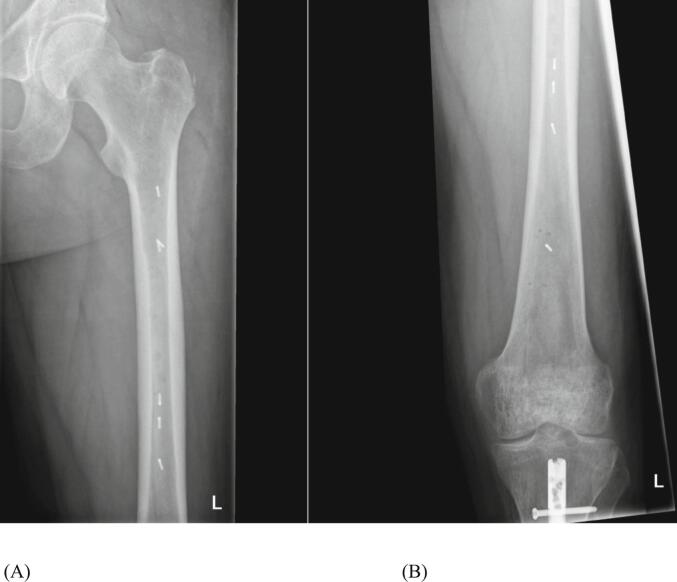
Postoperative radiographs of small metal debris parts of the broken reamer head in the intramedullary canal (A) and in the supracondylar region (B).
